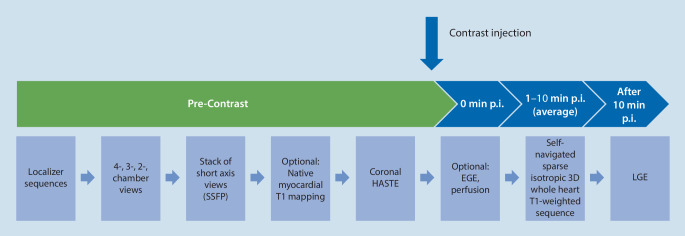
Sequence protocol overview for whole-heart CMR. *CMR* cardiovascular magnetic resonance imaging, *HASTE* half-Fourier single-shot turbo spin-echo sequence, *EGE* early gadolinium enhancement, *SSFP* steady-state free precession, *LGE* late gadolinium enhancement, *p.i.* post-injection
